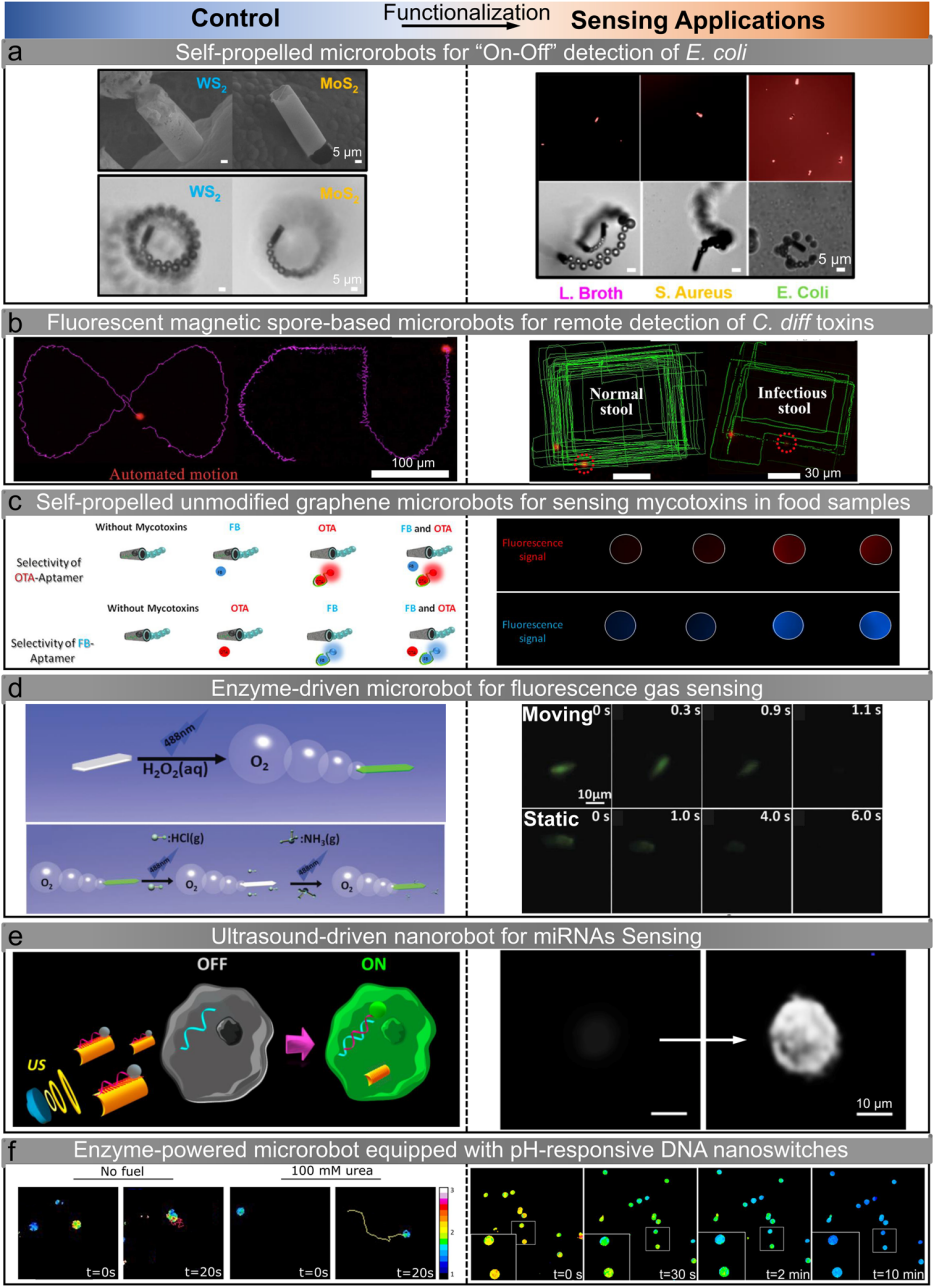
Self-generated signal-based sensing. a Peptide-modified self-propelled WS_2_/Pt and MoS_2_/Pt microrobots for on–off detection of *E. coli* [56]. Copyright (2020) American Chemical Society. b Fluorescent magnetic spore-based microrobots for remote detection of *C. diff* toxins [57]. Copyright (2020) The Authors. c Self-propelled unmodified graphene microrobots for quantitative analysis of mycotoxins in food samples [52]. Copyright (2017) American Chemical Society. d Enzyme-driven microrobot for fluorescence HCl and NH_3_ gases sensing [58]. Copyright (2016) Royal Society of Chemistry. e Ultrasound-driven nanorobot for single cell real-time miRNAs sensing [59]. Copyright (2015) American Chemical Society. f Self-sensing enzyme-powered microrobot equipped with pH-responsive DNA nanoswitches [60]. Copyright (2019) American Chemical Society
